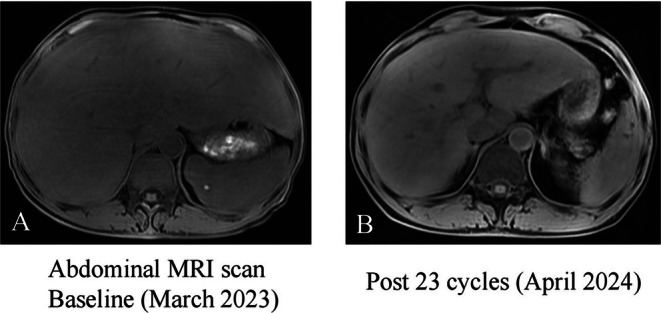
(A) Magnetic resonance (MR) imaging shows giant hepatomegaly prior to therapy initiation (baseline, March 2023). (B) Follow‐up MR imaging after 23 treatment cycles (April 2024) demonstrates marked reduction in liver size.
